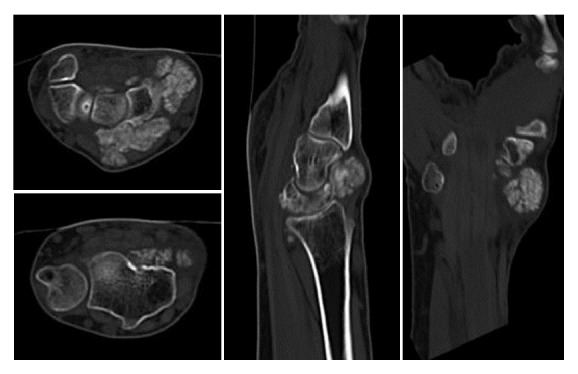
Computed tomography shows the details of the lesions.
